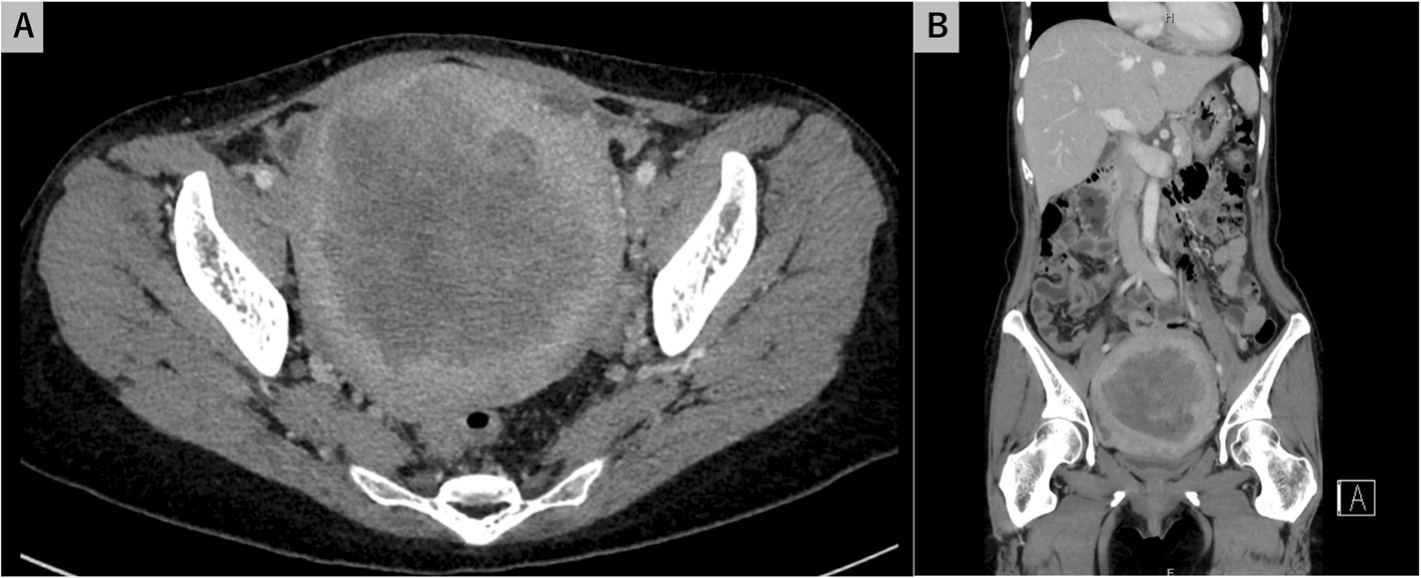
Contrast-enhanced computed tomography (CT) of the uterus after hospital admission. Although uterine enlargement was visible, no abscess formation was detected. a horizontal view; b coronal view
